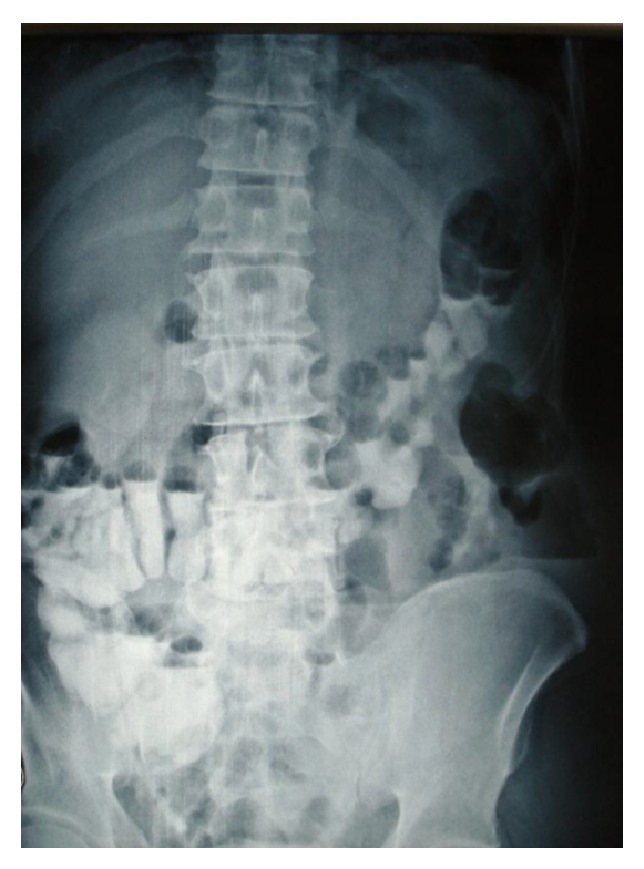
Abdominal X-ray showing inflammation at the left lumbar area.
